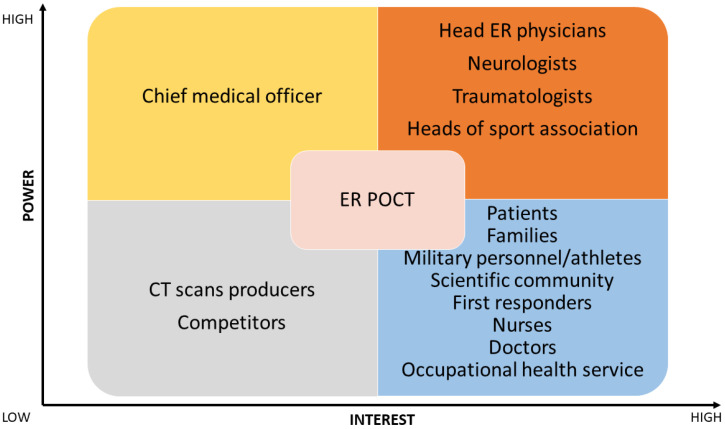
Stakeholder matrix for introducing biomarker testing as a point-of-care testing (POCT) in emergency rooms (ER). CT, computed tomography.
